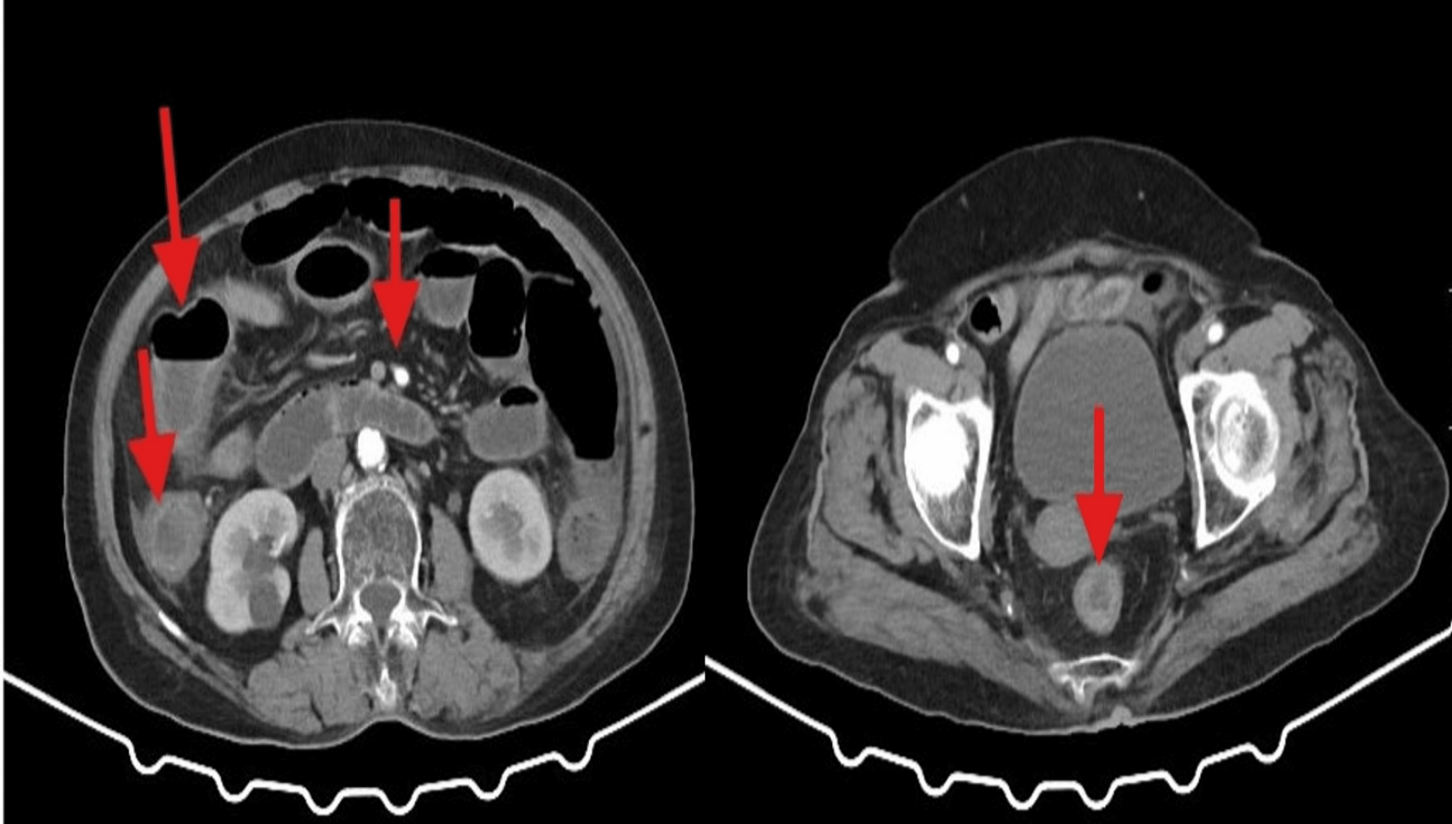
Axial view computed tomography with intravenous contrast Multiple calcified plaques in the superior mesenteric artery, thickening and edema of the intestinal walls of the ascending colon and transverse colon, incomplete low-position small intestinal obstruction, and thickening of the intestinal wall in the lower segment of the rectum (indicated by the red arrow).
